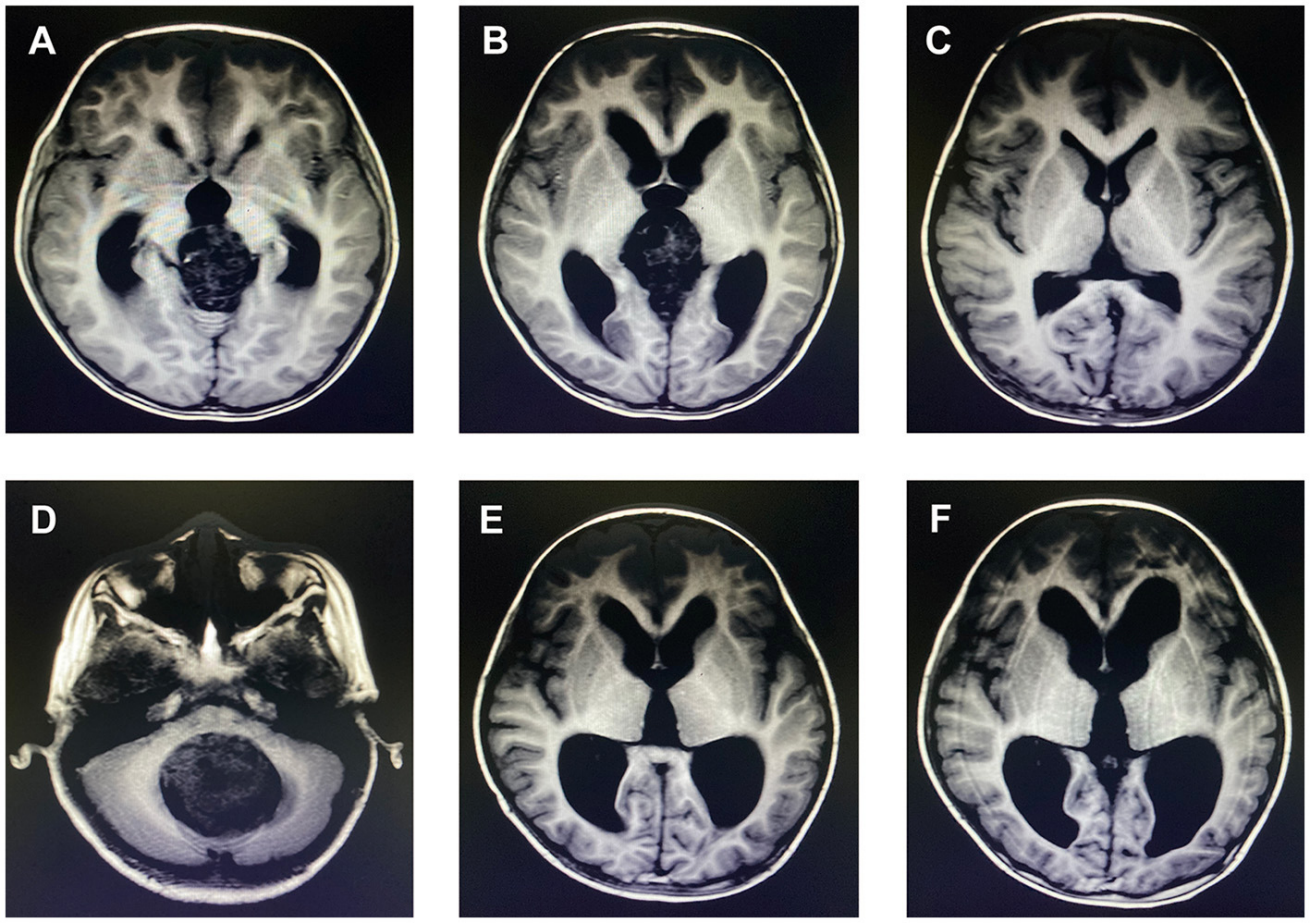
Patient 1. male, 3-year-old, immature teratoma in the third ventricle, volume: 3.8×3.2×3.5 cm, underwent preoperative Ommaya reservoir placement and then gross total resection. No symptoms and signs of increased intracranial pressure after surgery. (A) Imaging manifestation of tumor from preoperative MRI. (B) Imaging manifestation of enlarged lateral ventricle from preoperative MRI. (C) Imaging manifestation of lateral ventricle from MRI 3 months after tumor resection, it showed the enlarged ventricle returned to normal size. Patient 2. female, 7-year-old, medulloblastoma in the fourth ventricle, volume: 5.0 × 4.9 × 5.2 cm, underwent preoperative EVD and then gross total resection. Symptoms like headache, vomiting, ataxia were seen after surgery. (D) Imaging manifestation of tumor from preoperative MRI. (E) Imaging manifestation of enlarged lateral ventricle from preoperative MRI. (F) Imaging manifestation of lateral ventricle from MRI 3 months after tumor resection, it showed the persistent enlarged ventricle.
